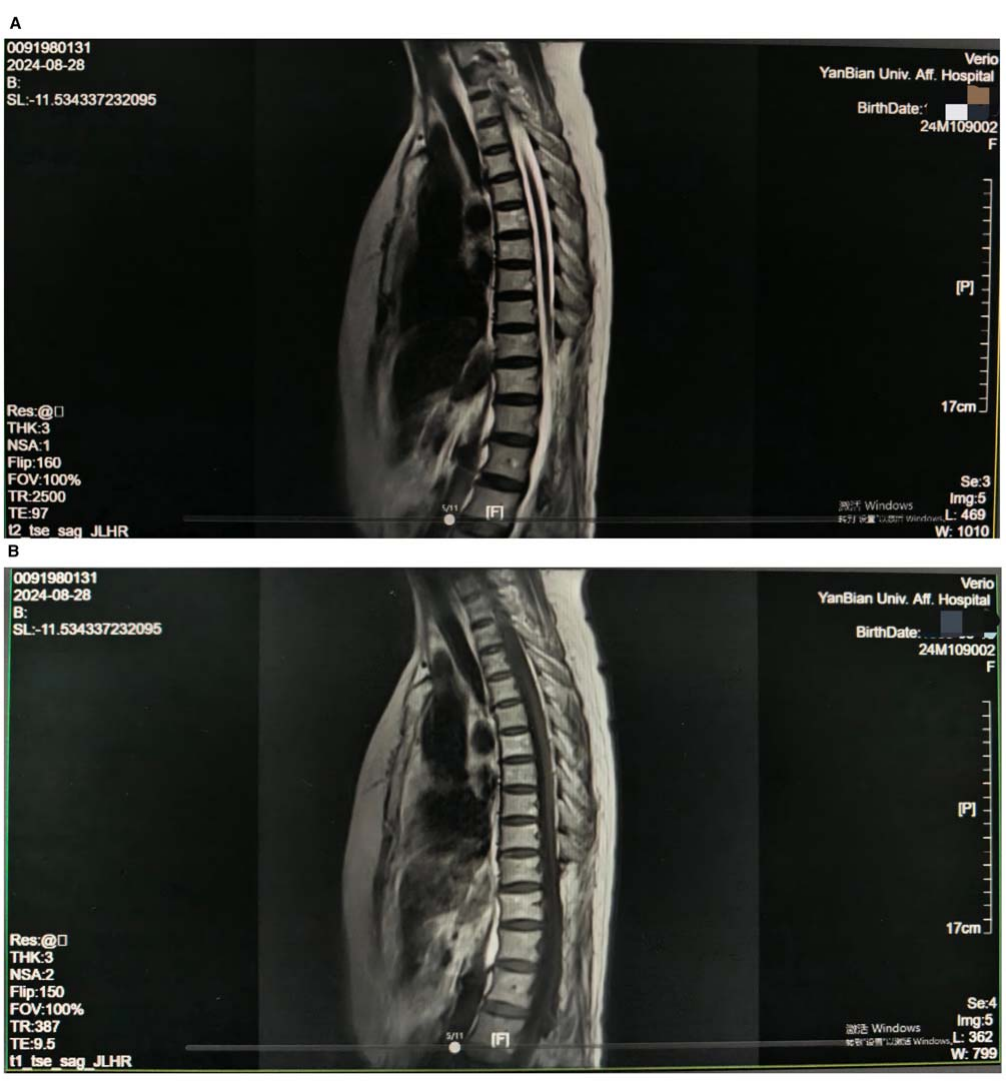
(A, B) Pre-operative spinal MRI from this admission, showing post-operative changes in the thoracolumbar region with absent spinous processes and laminae at T10, T11, T12, L1, and L2, along with heterogeneous signals.
